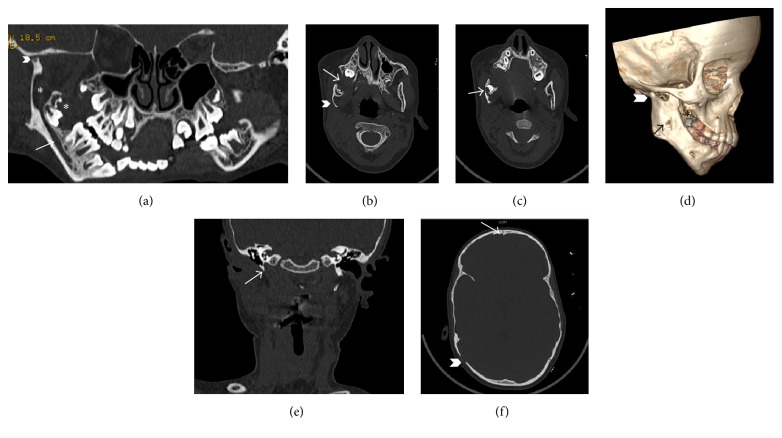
(a)* Panoramic dental scan* depicting enlarged inferior alveolar canal (arrow) with residual tumor mass (asterisk) and hypoplastic condylar process (chevron), (b)* axial CT* in bone window showing the residual mass in alveolar margins of maxilla and mandible (arrow) and concavity of mandibular ramus (chevron), (c)* axial CT scan* showing apparent foramen (arrow) due to lytic erosion of mandibular cortex, (d)* 3D CT scan* image showing hypoplastic condylar process (chevron), apparent foramen (arrow), and tumor in maxillary alveolus (asterisk), (e)* coronal CT* image showing rudimentary styloid process of right side (arrow), and (f)* axial CT* image of sutural diastasis (chevron) and persistent metopic suture (arrow).
